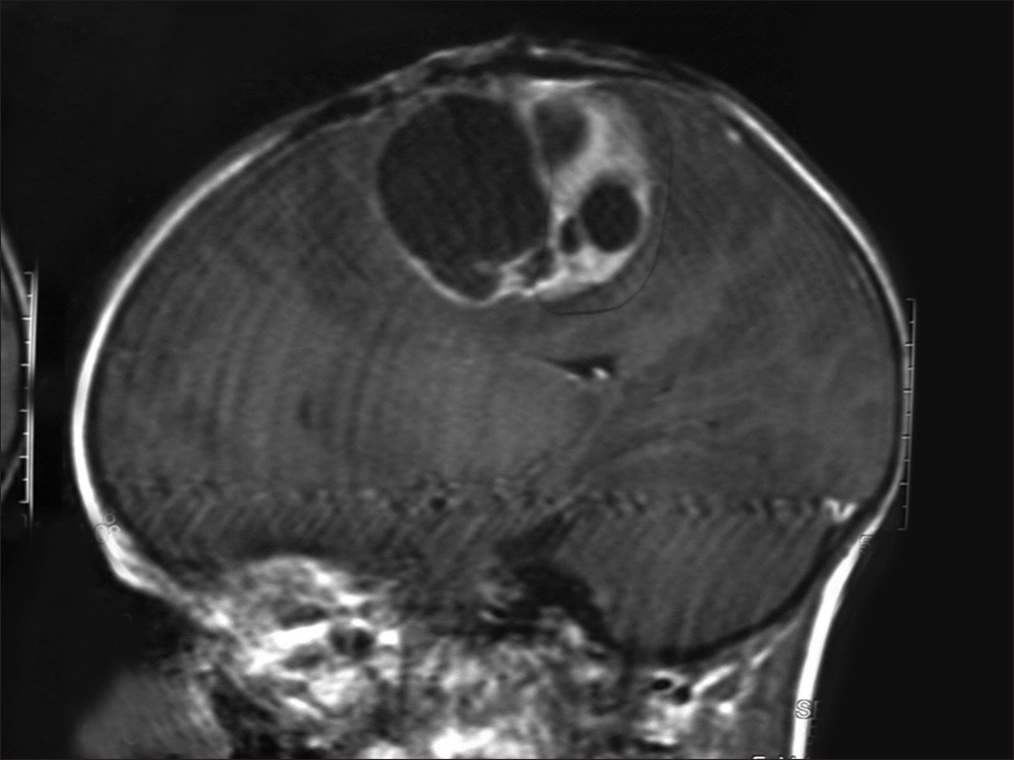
Saggital MRI brain with a glioblastoma multiforme in a male farm worker
